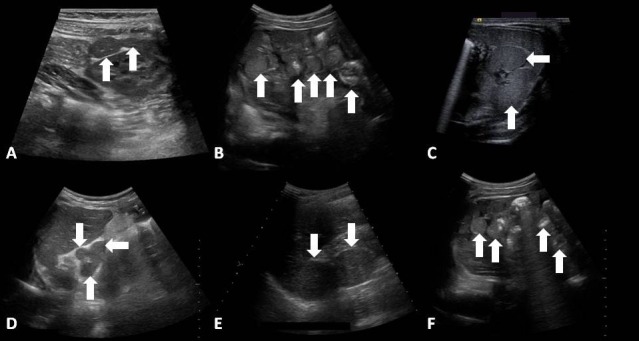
Splenic anatomy by ultrasound Abdominal ultrasound imaging from six different patients demonstrating polysplenia. Splenic tissue in the setting of heterotaxy can be found in various parts of the abdomen with a varying number of spleens.
